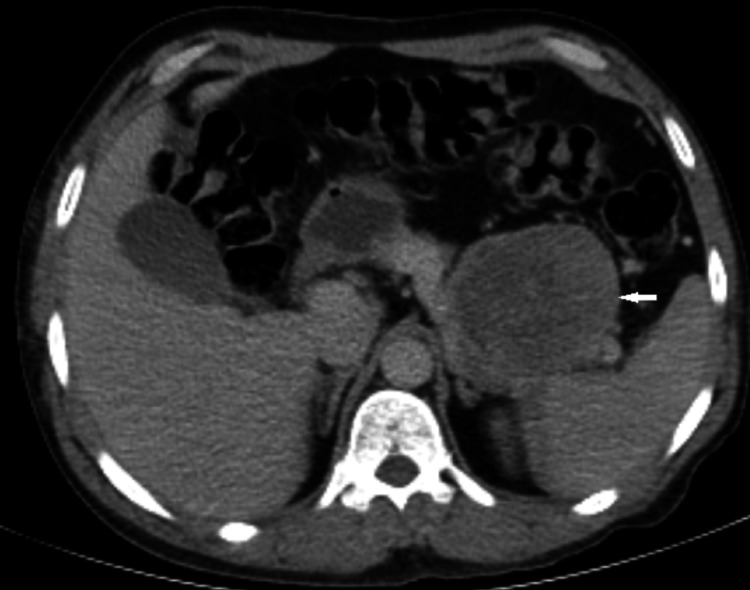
Pre-operative axial CT section at upper abdomen showing an enhancing mass in the tail of the pancreas
